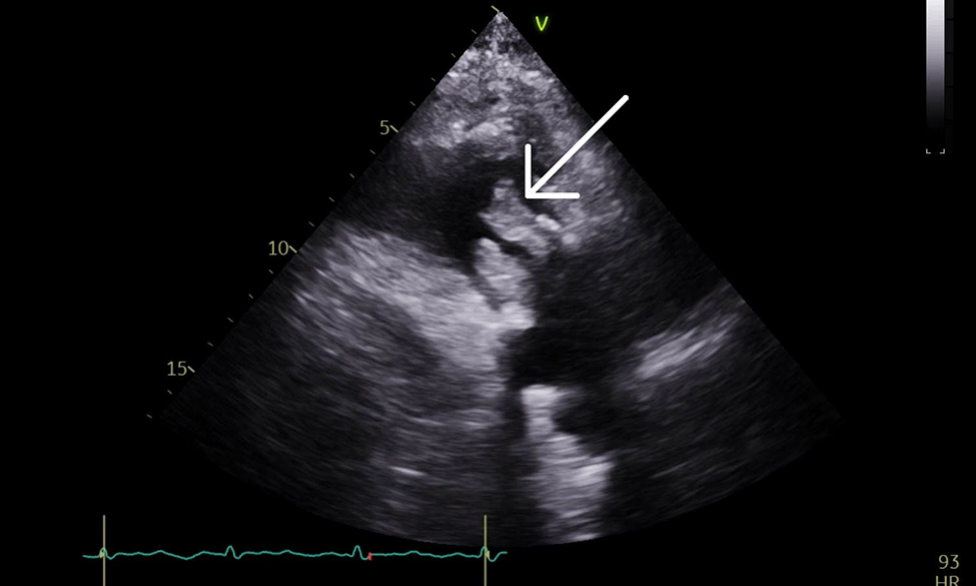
Echocardiogram of patient from case report of *Aerococcus urinae* tricuspid valve endocarditis. Arrow indicates tricuspid mass. V at top of image indicates positive inflection. Numbers on the left side indicate depth from the ultrasound probe (in cm). Electrical signal profile is indicated at the bottom of the image.
